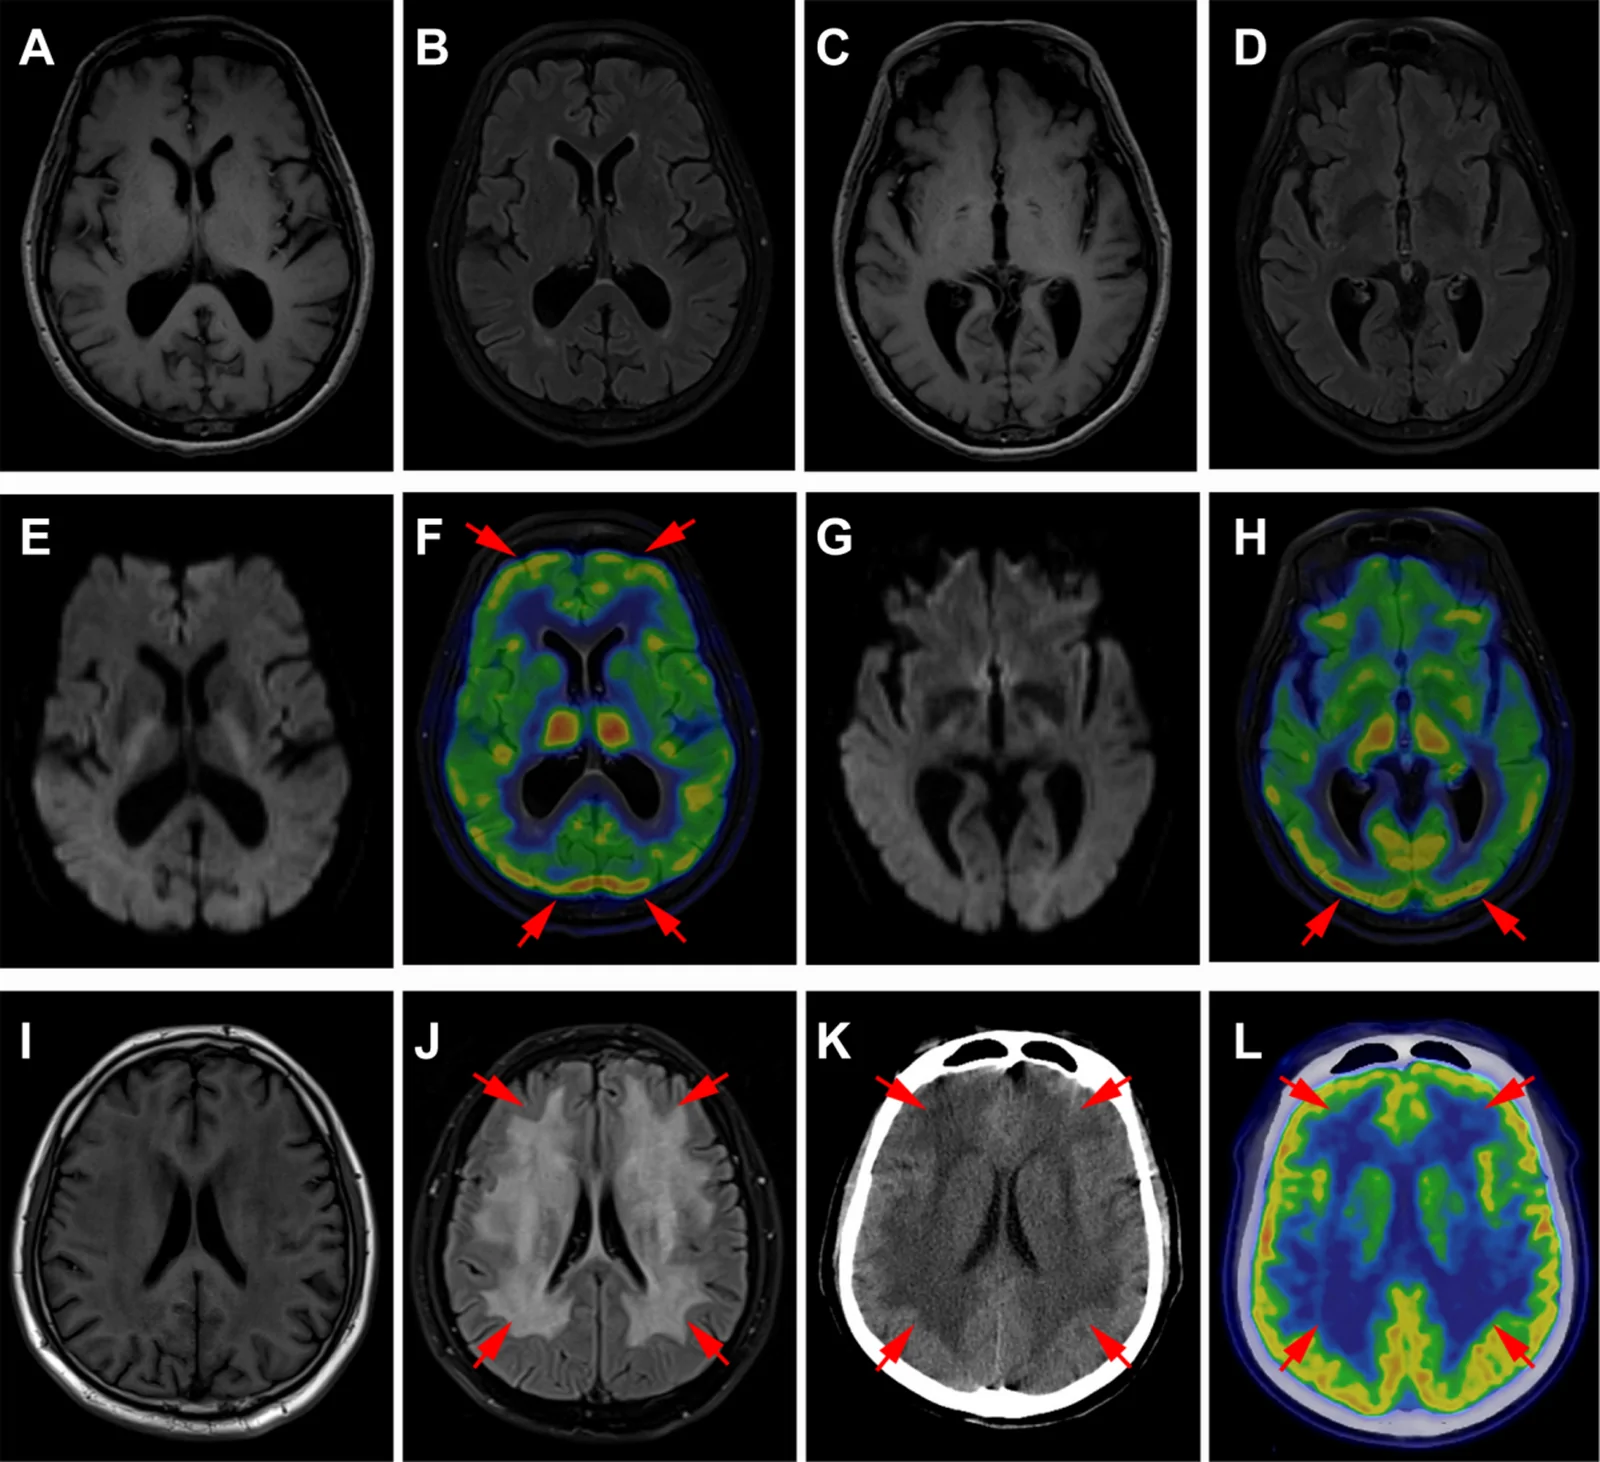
Imaging results of patients 2 and 3. (A–H) ^18^F-DPA-714 PET/MRI of Patient 3, showing increased tracer uptake in the frontal and occipital lobes (F and H), with no obvious abnormalities on T1 (A and C), T2-FLAIR (B and D), or diffusion-weighted imaging (E and G). (I–J) Brain MRI of Patient 2, demonstrating slightly low T1 signal and significantly high T2-FLAIR signal in the bilateral white matter. (K–L) ^18^F-FDG PET/CT of Patient 2, showing extensive low-density on CT (K) and diffuse reduction in ^18^F-FDG uptake in the bilateral white matter (L)
